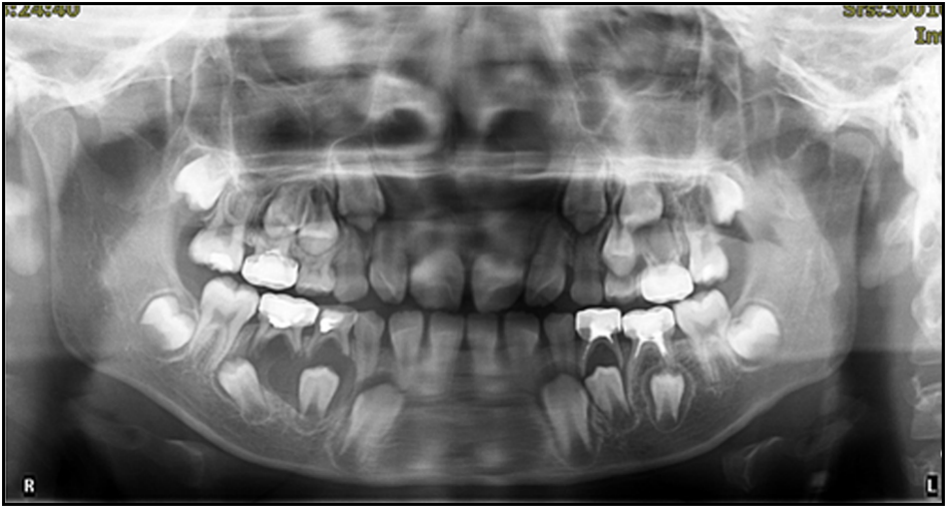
Severe periapical infection of lower right primary teeth caused displacement of lower right 2nd premolar, inducing ectopic eruption
